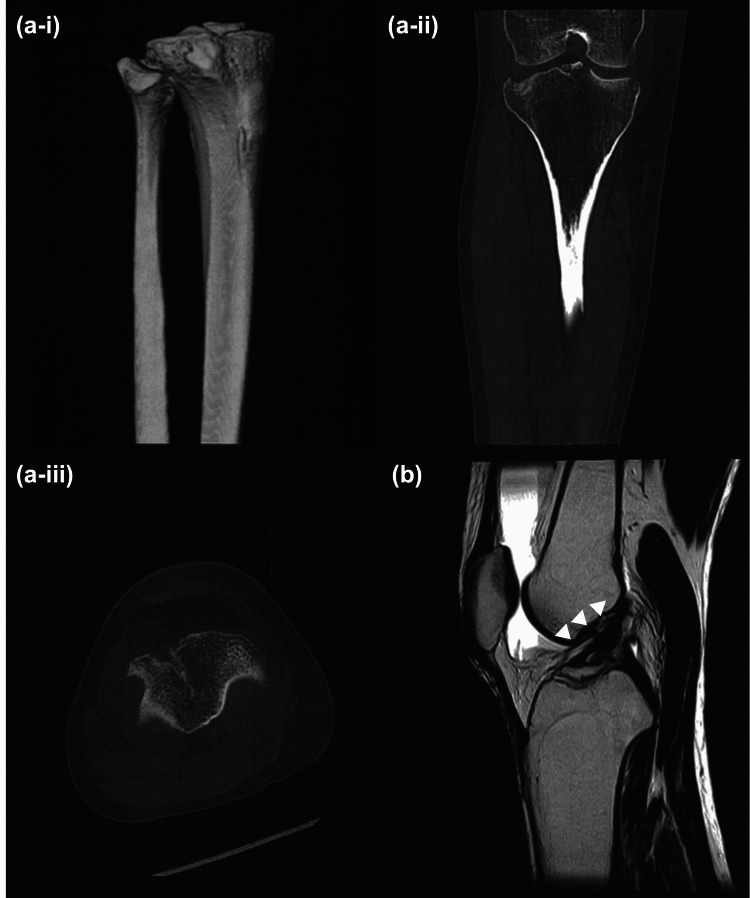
Radiological findings of the tibial plateau fracture (a) Computed tomography (CT) images at the time of injury: (a-i): Three-dimensional CT. (a-ii): Coronal plane. (a-iii): Transverse plane. (b) Magnetic resonance imaging (MRI) image at the time of injury: Suspicion of the anterior cruciate ligament injury.
